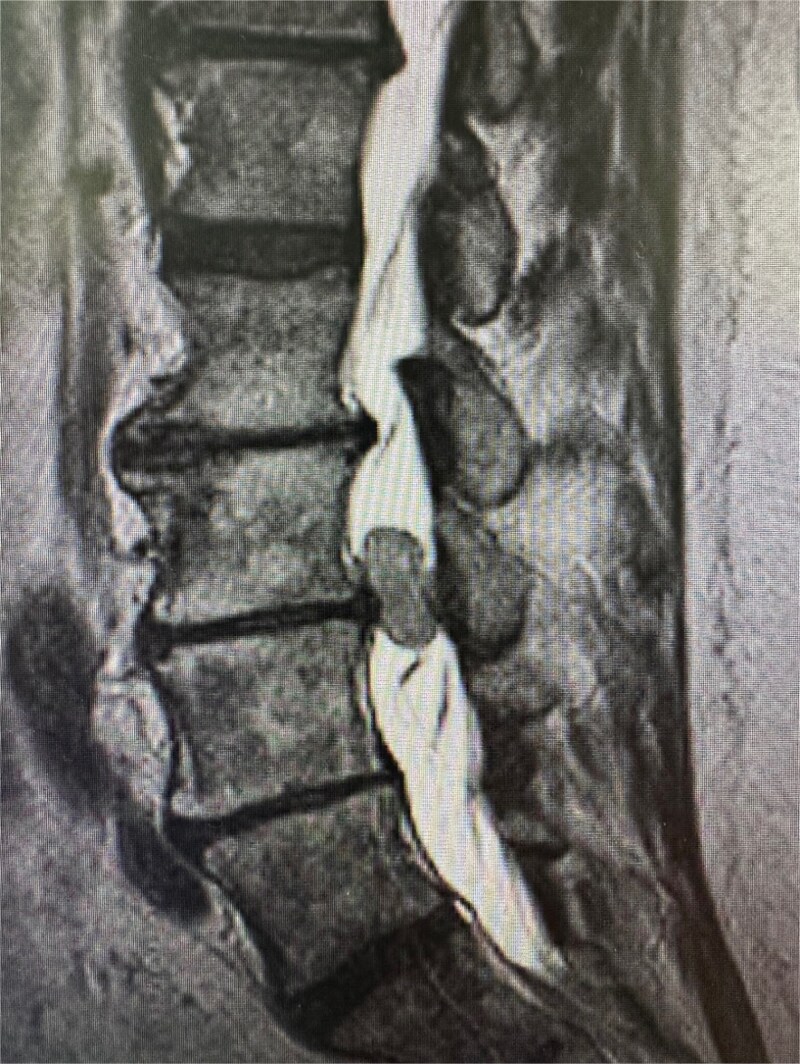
Sagittal T2-weighted MRI of the lumbar spine demonstrating a well-circumscribed intradural extramedullary mass measuring 0.9 × 1.4 × 1.9 cm at the L3–L4 level. The lesion exhibits intermediate signal intensity.
